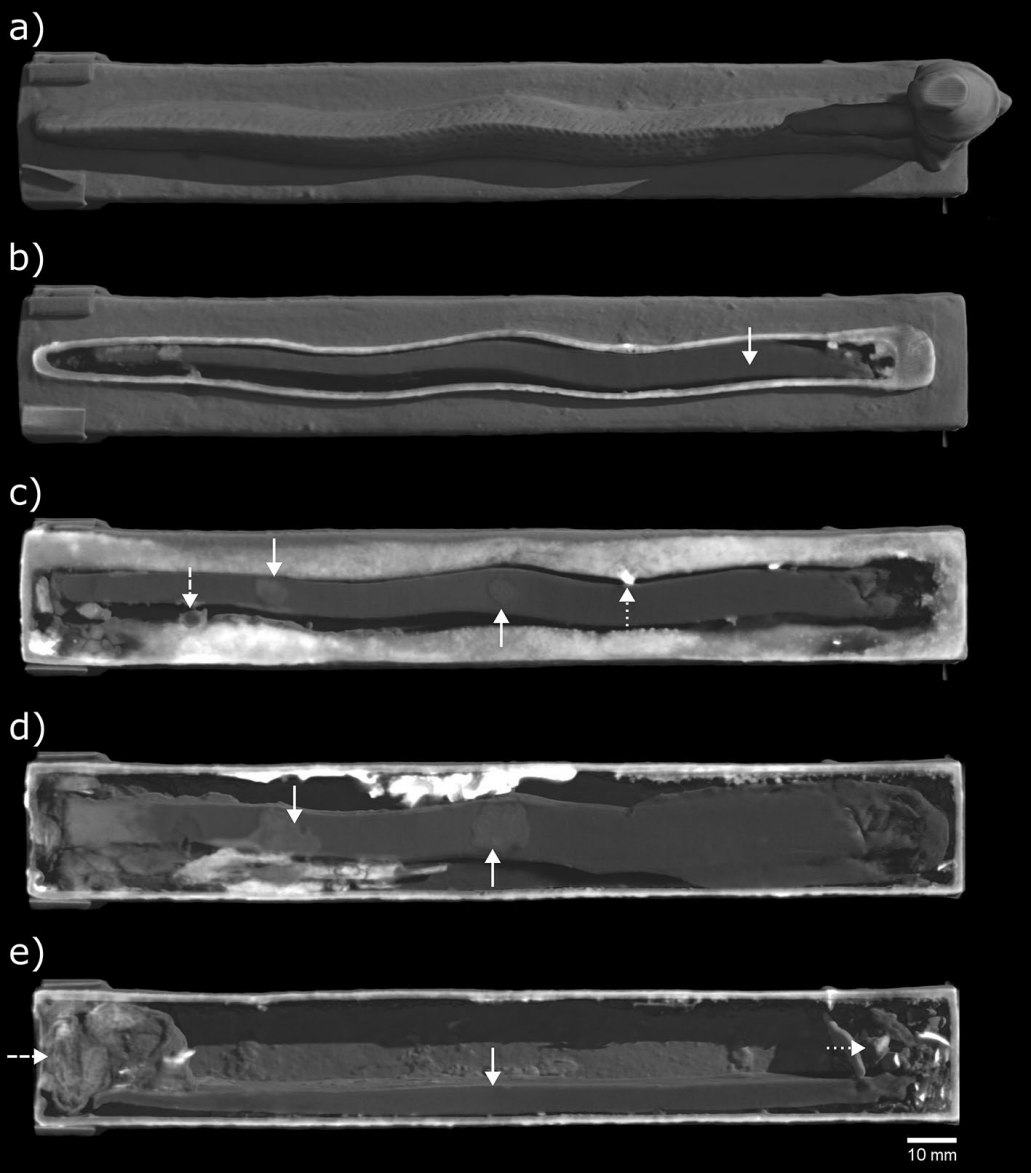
EA71428 neutron CT volume renders. (a) complete view of box; (b)–(e) cutaway views at different heights (measured from the box base): (b) 24.0 mm, through eel figure (solid arrow: upper lead piece inside hollow); (c) 21.3 mm, through top box wall (solid arrows: lead corrosion, dashed arrow: repair to box, dotted arrow: chaplet); (d) 17.7 mm, through base of upper lead piece (solid arrows: lead corrosion); (e) 12.9 mm, through box hollow (solid arrow: lower lead piece, dashed arrow: textile in plug, dotted arrow: loose fragments).
